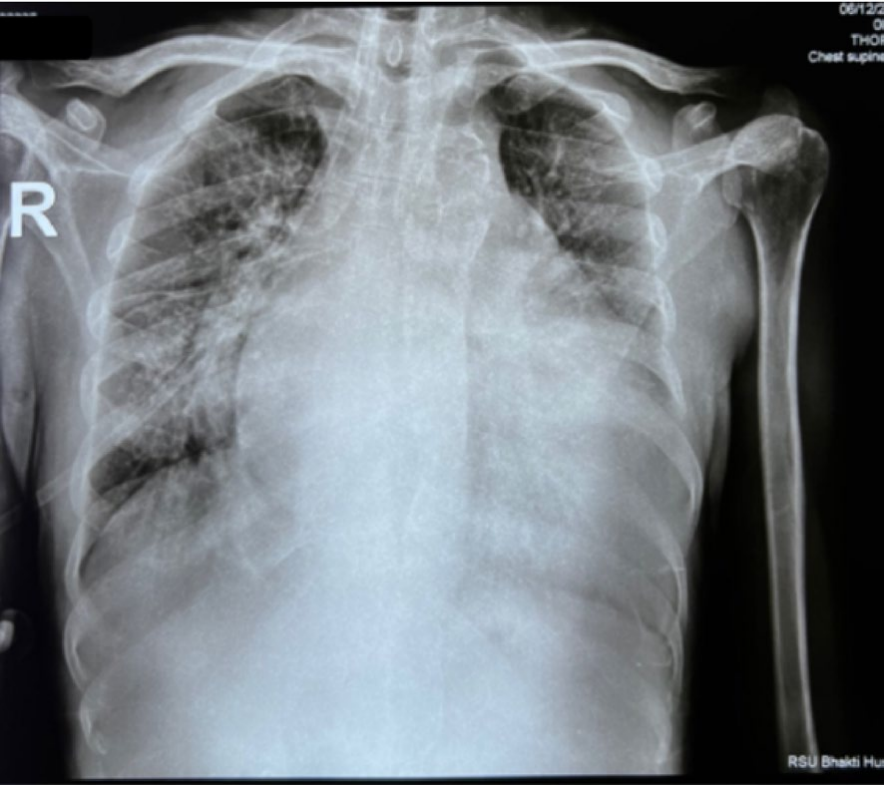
Chest X-ray demonstrating significant cardiomegaly, characterized by an enlarged cardiac silhouette, suggesting advanced cardiac involvement
